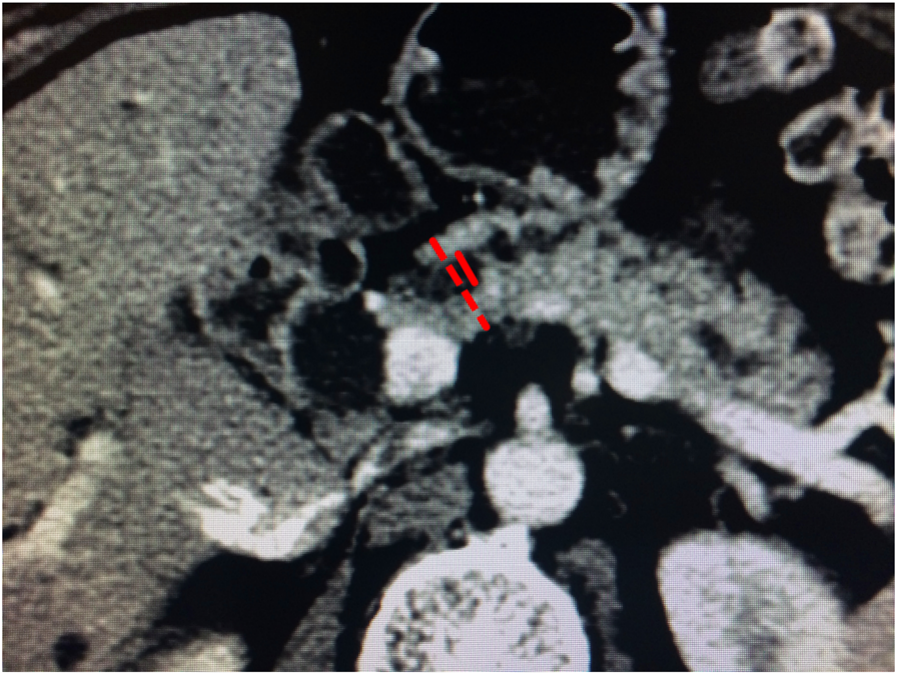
Diameter of the main pancreatic duct (MPD) (D) and thickness of the pancreas (T) were measured on enhanced computed tomography images. Preoperatively, the values were measured along the line at the left edge of the superior mesenteric vein (SMV). Postoperatively, the parameters were measured at the same area in the remnant pancreas. The degree of pancreatic atrophy was calculated by the results of T – D. Note: Dotted line indicates the thickness of the pancreas; continuous line indicates diameter of the main pancreatic duct
